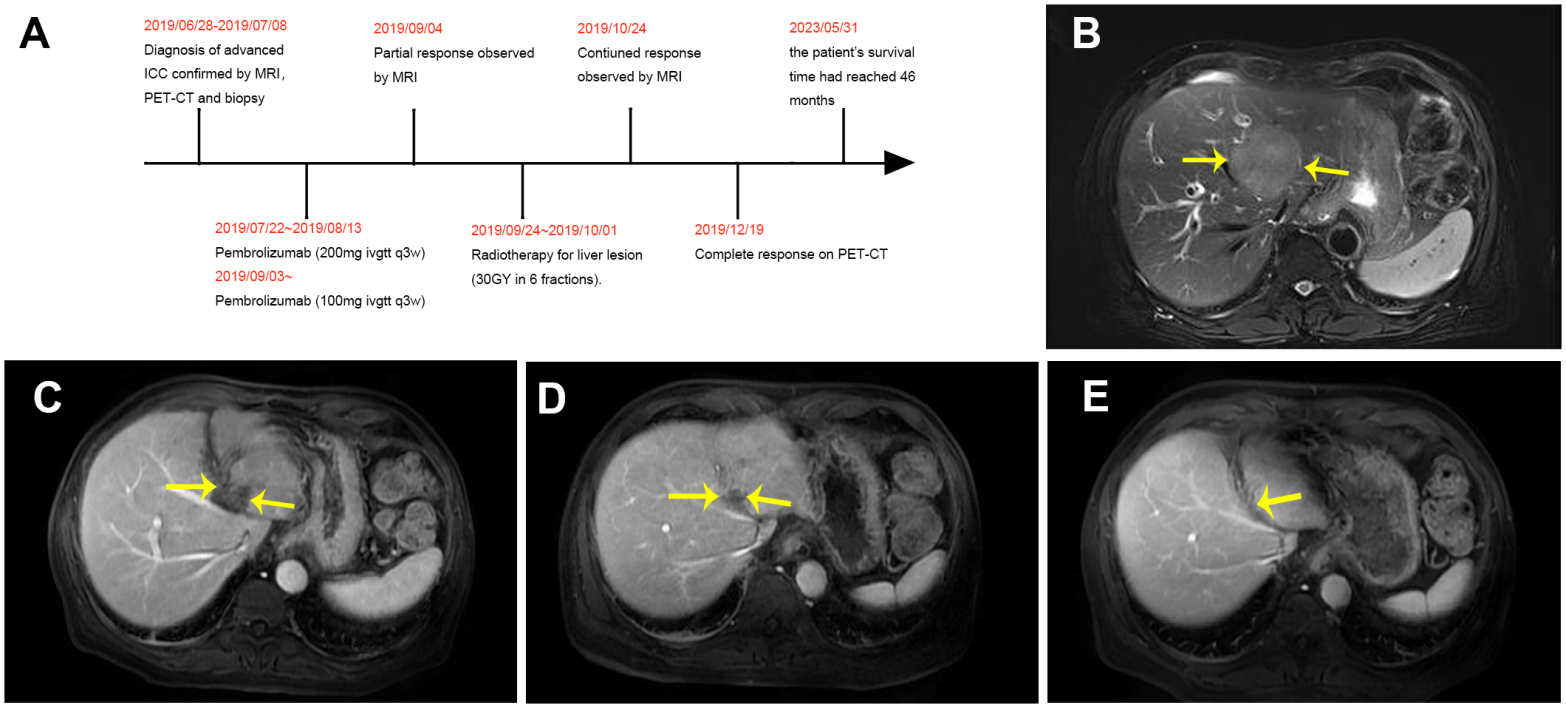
Timeline and MRI examination. Yellow arrows indicate the changes in lesion size. (A) Timeline of initial diagnosis, anti-PD-1 immunotherapy, radiotherapy and follow-up. (B) MRI results (June 28, 2019) prior to therapy. (C) MRI results (September 4, 2019) following 3 cycles of anti PD-1 treatment. (D) MRI results (October 24, 2019) following anti-PD-1 immunotherapy in combination with radiotherapy. (E) MRI results (April 8, 2020) following anti-PD-1 immunotherapy maintenance therapy.
